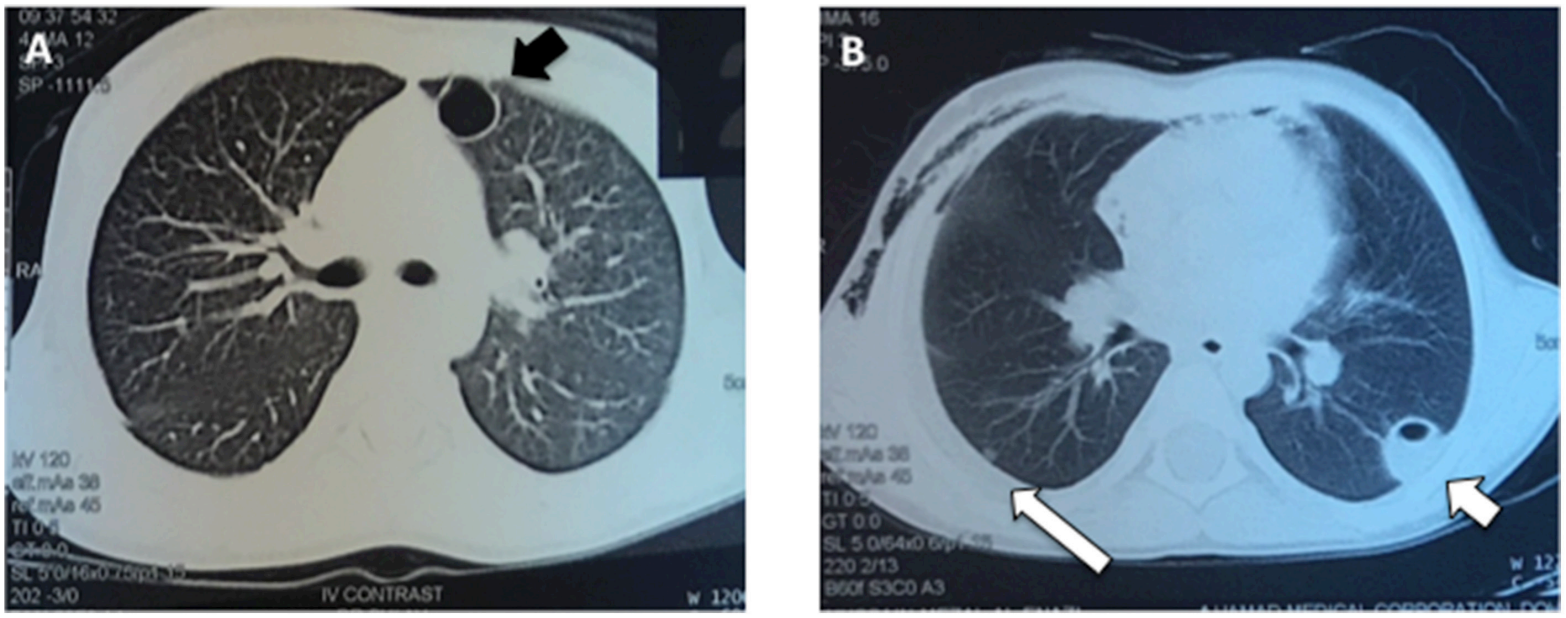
Pulmonary imaging during an acute respiratory infection at age 5 years. (A) The patient had numerous, enhancing cavitary lung lesions (black arrow) noted using CT scan of the chest, which improved after drainage and prolonged antibiotic therapy. (B) Several of the pneumatoceles were fluid-filled (short white arrow) and a parapneumonic effusion was present (long white arrow).
